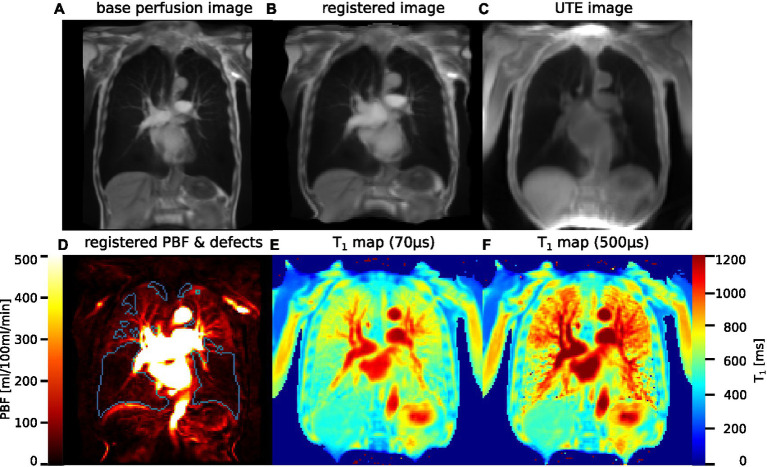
(A) Original image from DCE MRI, averaged over all timepoints and three slices. (B) The same image, registered to the UTE image. (C) Image derived from inversion recovery UTE that was used as registration target. (D) PBF map after application of the distortion field produced by the image registration, with perfusion defects delineated in blue. (E) T_1_ map acquired using UTE at TE_1_ = 70 μs. (F) T_1_ map at TE_2_ = 500 μs.
